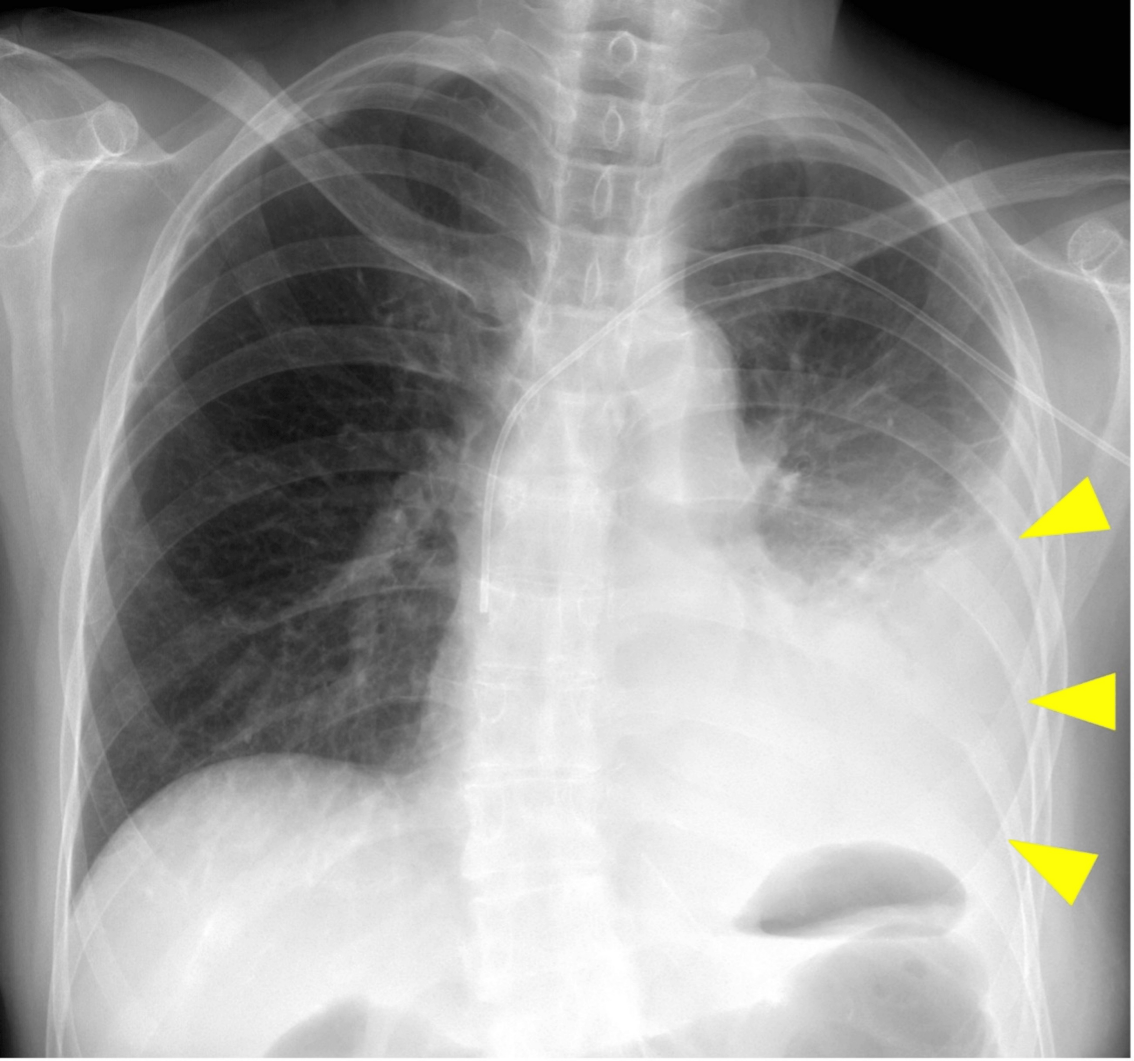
Chest X-ray revealing a large left pleural effusion (arrowheads)
